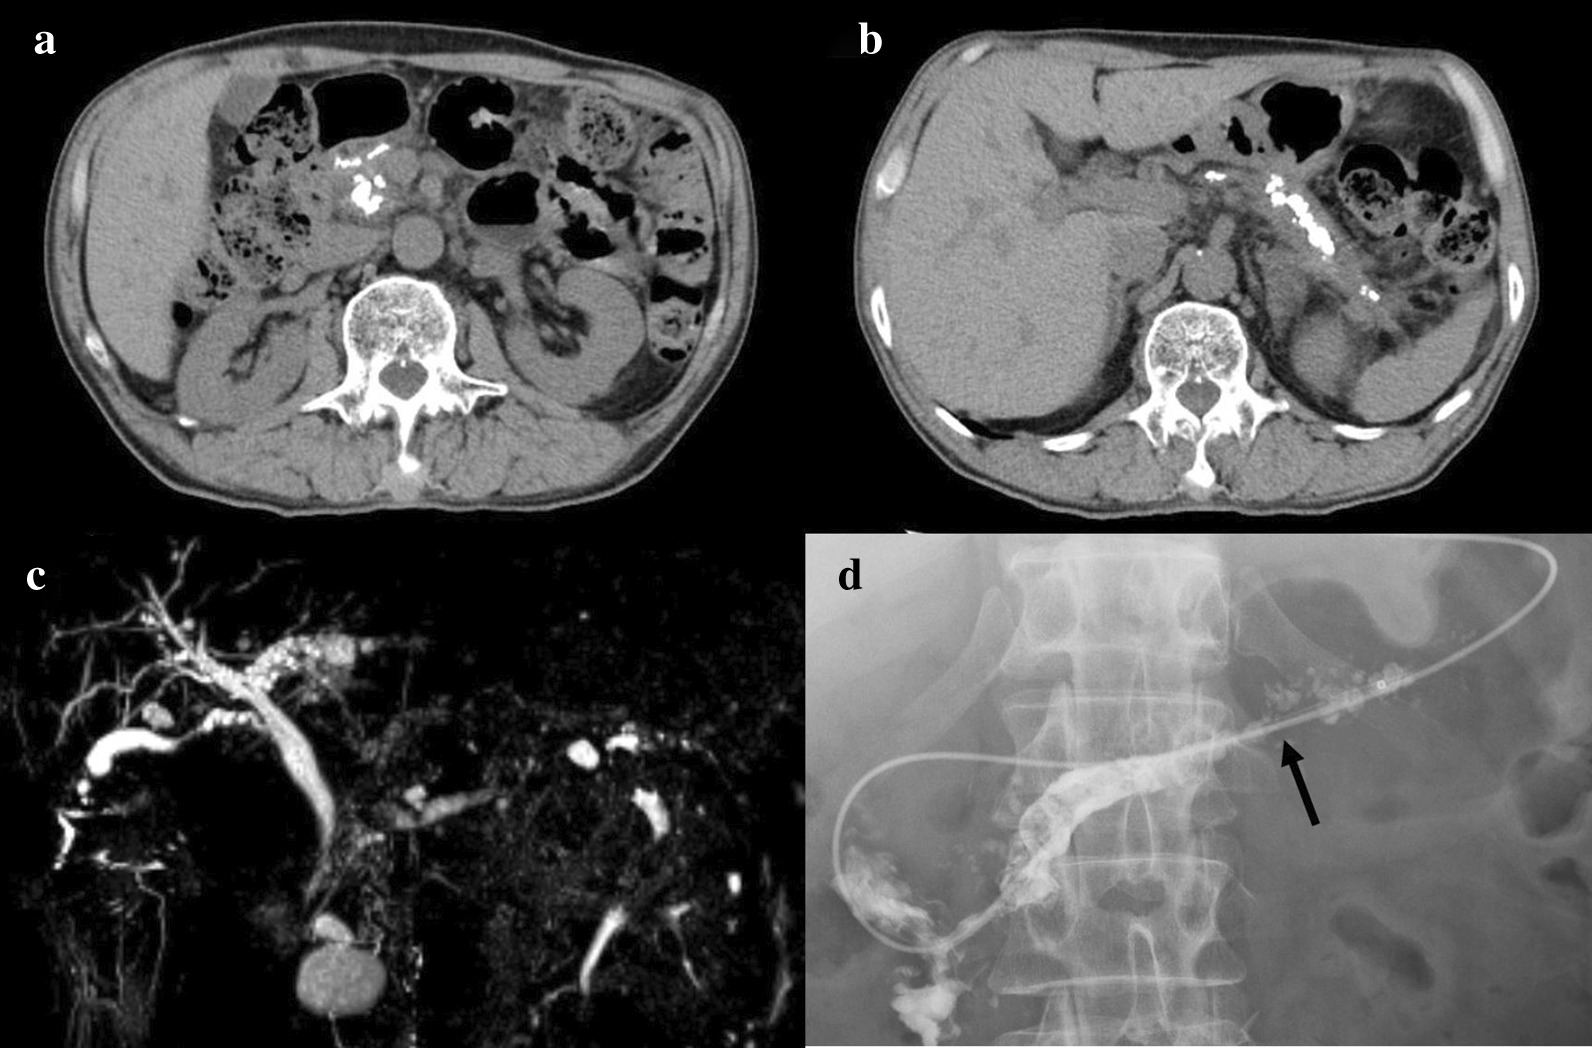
Representative images of the patient who underwent distal pancreatectomy and the Frey procedure for chronic pancreatitis with the hybrid approach. a, b Computed tomography images are shown. c Magnetic resonance cholangiopancreatography is shown. d Endoscopic retrograde cholangiopancreatography is shown. Main pancreatic duct was severe stenosis (arrow)
